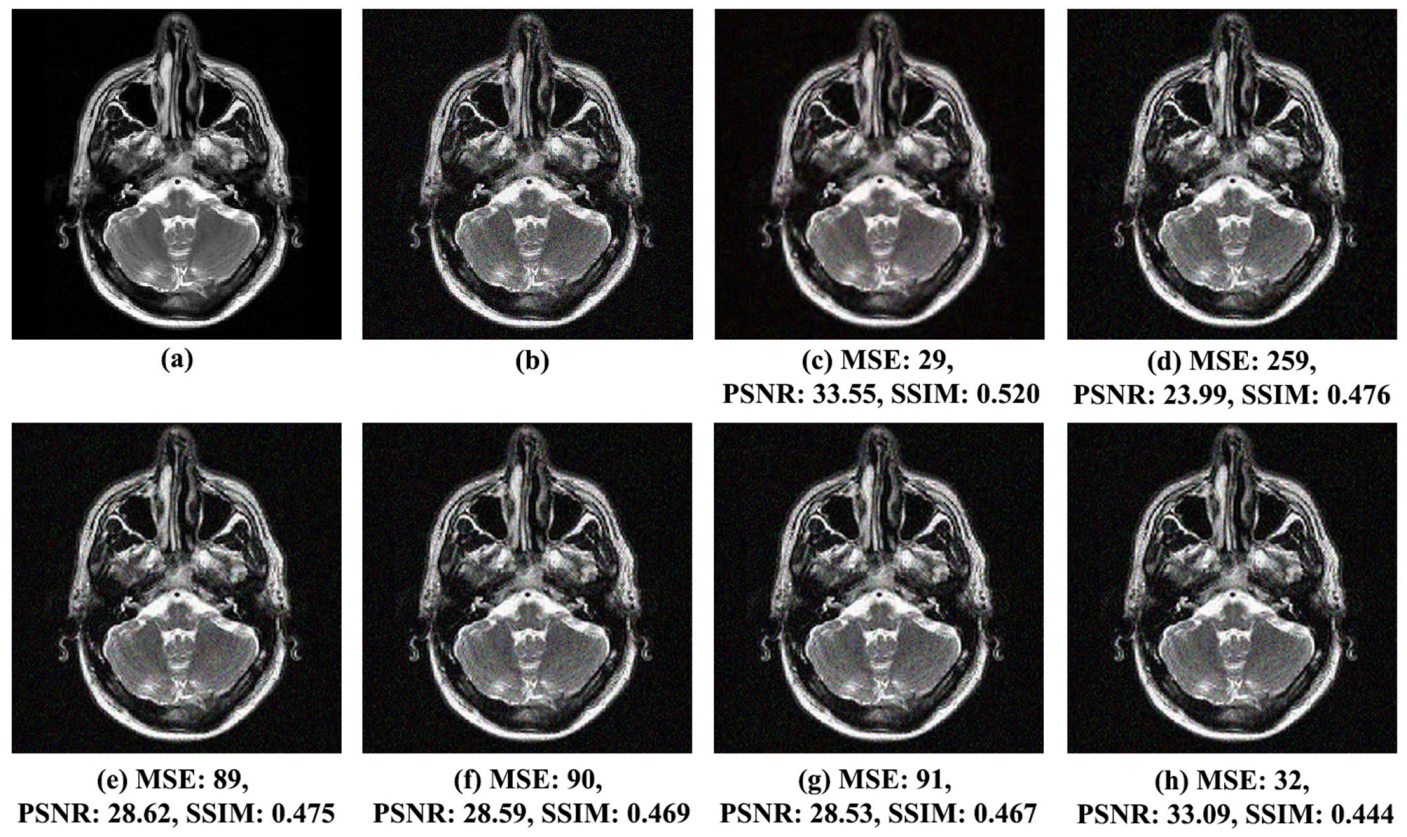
Comparison of noise reduction performance for MRI Brain Image 1 with noise variance of 0.01: (A) original image, (B) noisy image, (C) proposed method, (D) VisuShrink, (E) BayesShrink, (F) Gaussian Kernel, (G) improved threshold, and (H) AGGD.
